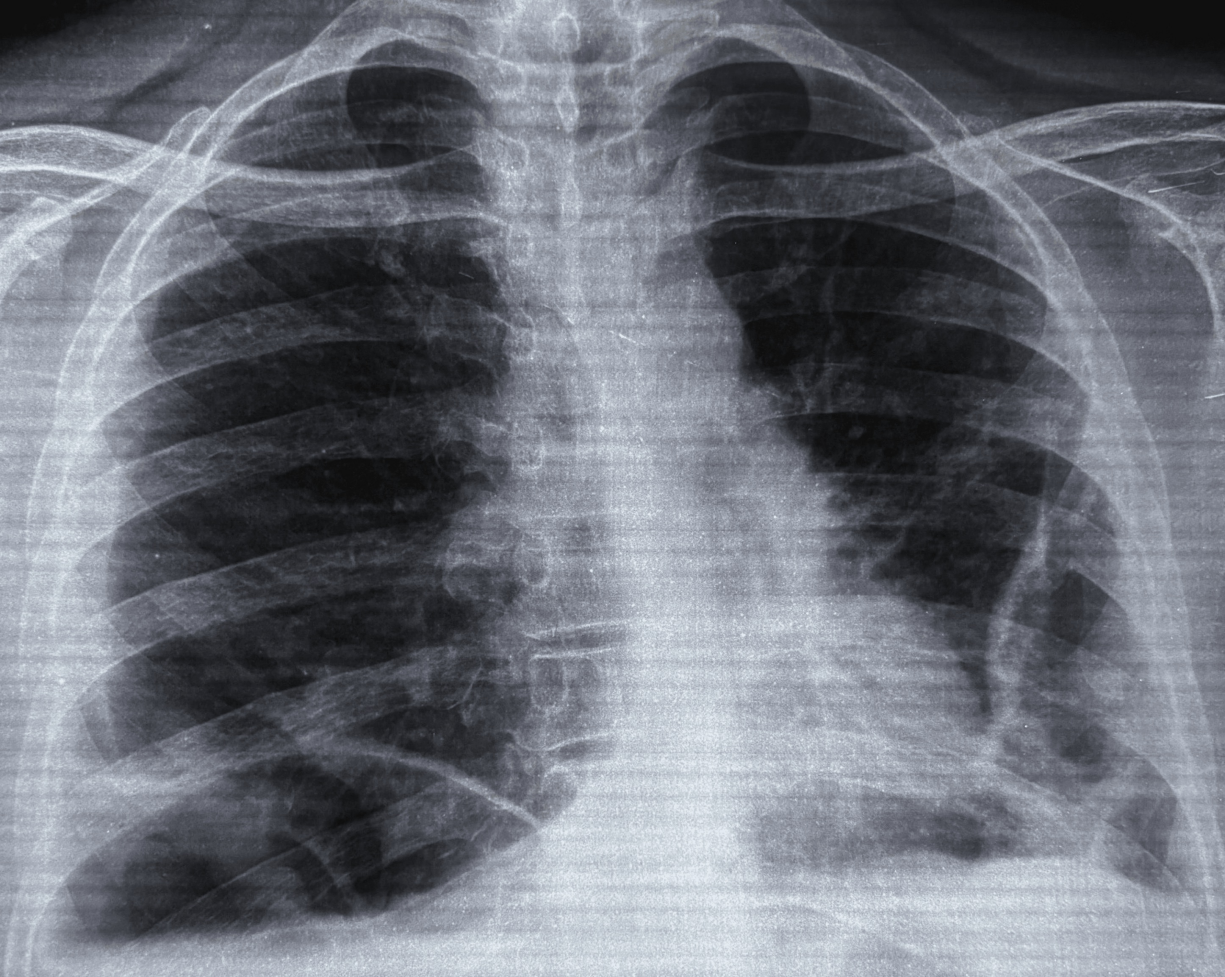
Chest X-ray of the patient showing obliteration of bilateral costophrenic angles with an air fluid level in the right lower zone
